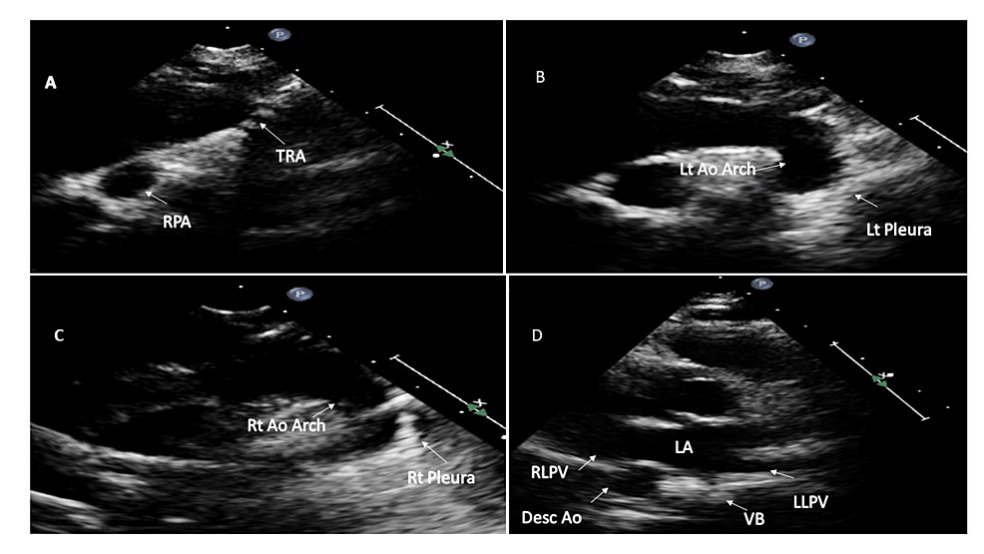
Sequential two-dimensional echocardiographic images of double aortic arch (A) Suprasternal long axis sweep with the probe position at 12 o'clock shows tracheal cartilages (TRA). (B) Tilting the probe leftwards shows the left aortic arch (Lt Ao Arch) and left pleura (Lt pleura). (C) Tilting the probe rightwards from 12 o'clock shows the right aortic arch (Rt Ao Arch) and right pleura (Rt pleura). (D) Right descending thoracic aorta (Desc Ao) in this patient with double aortic arch. RPA: right pulmonary artery; VB: vertebral body; RLPV: right lower pulmonary vein; LLPV: left lower pulmonary vein; RPA: right pulmonary artery; LA: left atrium.
